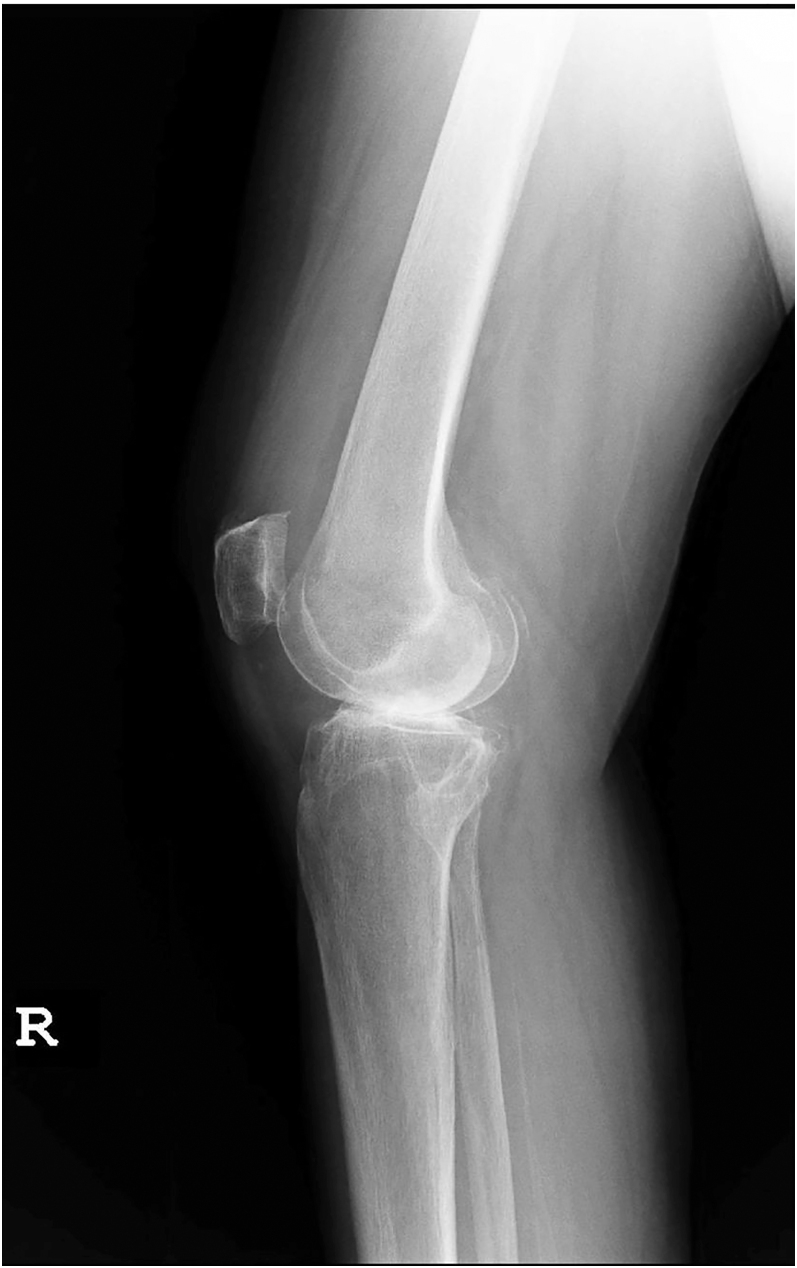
Sagittal plane imaging. Non-weight-bearing radiographs do not need to be full-length images.
